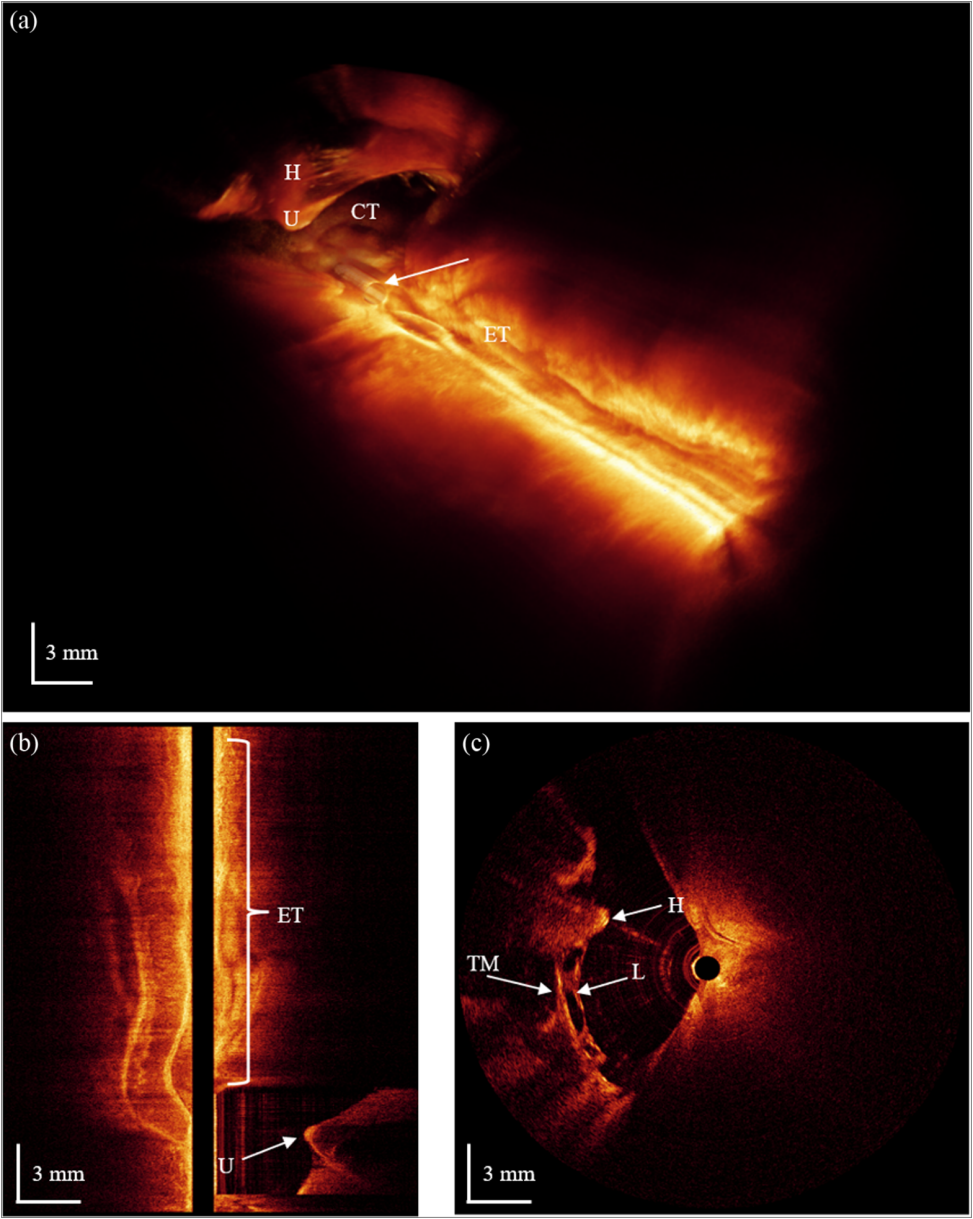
(a) Volumetric OCT image of the middle ear space taken with the catheter endoscope. Here, H is the handle of the malleus, U is the umbo of the tympanic membrane, CT is the chorda tympani, and ET is the Eustachian tube. The arrow indicates part of the catheter—seen here as a perfect cylinder. Panel (b) shows many layers of cartilage and fatty deposits along a cross-section of the Eustachian tube along with the umbo in the middle ear. Panel (c) is an orthogonal cross-section to panel (b) where the handle of the malleus (H) is seen with a lot of connective tissue (L), likely ligaments, attaching to it, the tympanic membrane (TM), and the attic wall of the middle ear.
